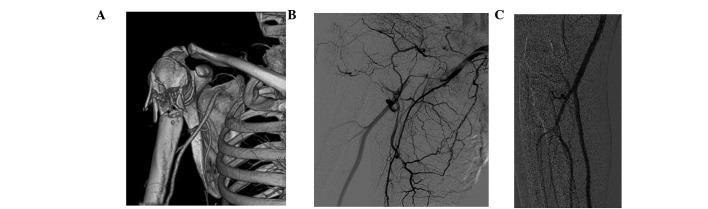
Broken humeral surgery neck and the injured axillary artery of case 3. (A) CTA of the right proximal humerus with a comminuted fracture of the surgical neck and the thinned, discontinued vessel of the first and second segments of the axillary artery. (B) DSA shows the incomplete axillary artery with extensive collateral circulation. (C) DSA shows the brachial, radial and ulnar arteries were engorged well. CTA, computerized tomography angiography; DSA, digital subtraction angiography.
